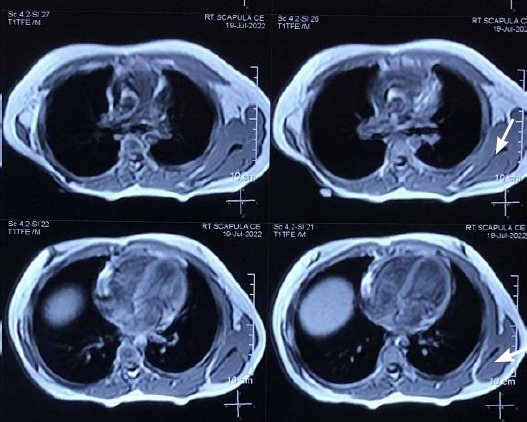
Follow-up MRI at 2 years showed no evidence of recurrence.
